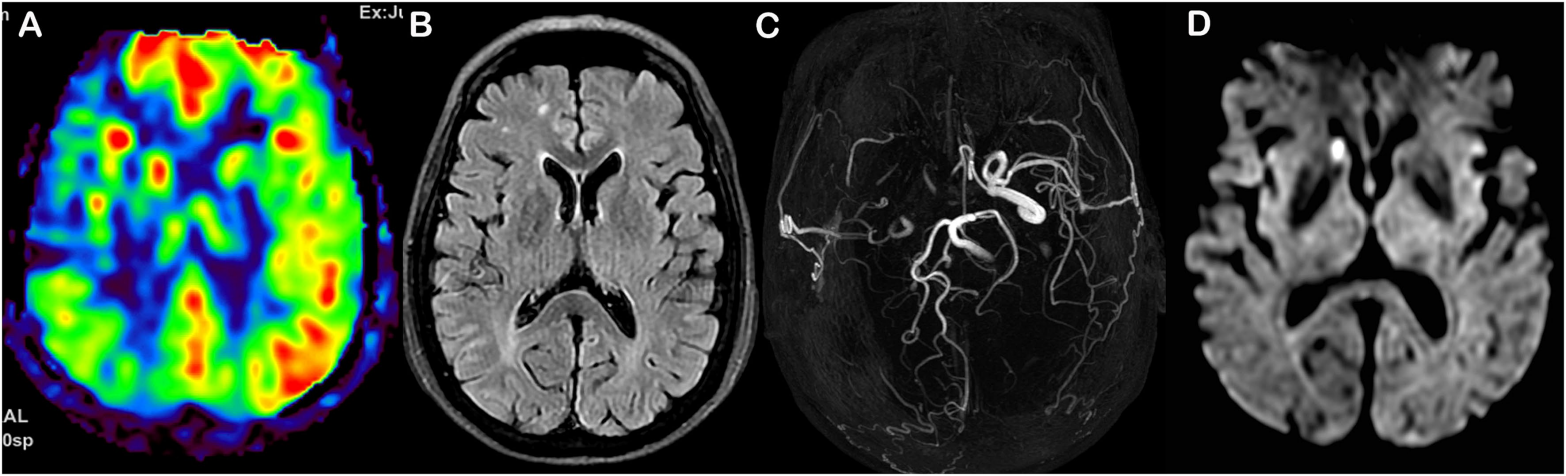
Representative MMD case with poor prognosis (A–D). Female, 60 years old, with an admission mRS score of 2, and an mRS score of 3 after 1 year. T2-FLAIR showing ivy sign (A); Preoperative ASL-ASPECT score was 4 (B). Houkin score was 6 at 1 week postoperatively, and MRA staging was stage 3 (C); DWI showing acute/subacute infarction in the right caudate nucleus (D). The nomogram score was 130, with a probability of poor prognosis of 0.90.
